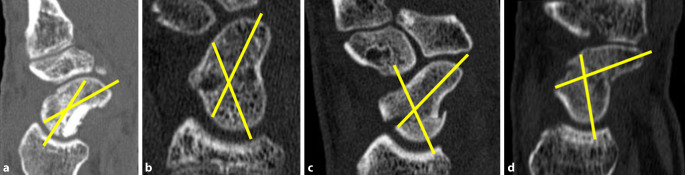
Um Messfehler und die Intraobserver-Variabilität zu reduzieren, wurden die CT am gleichen Tag vom gleichen Untersucher begutachtet.
